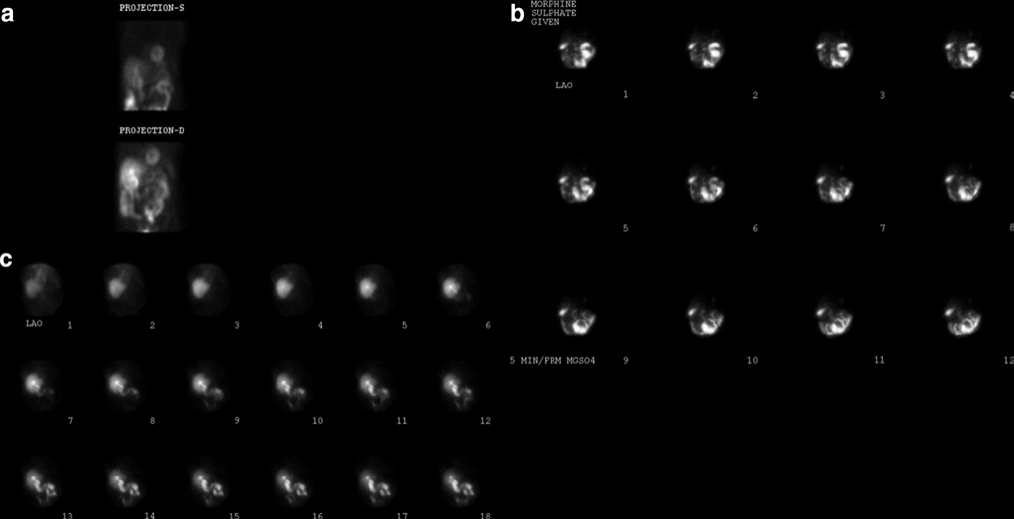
GB not visualized on the stress/rest MIBI (a) or HBS data (b), except post MS04 (c). *Incidentally, the MIBI, pre-MS04 and post-MS04 HBS studies were acquired in three different γ cameras*. GB, gall bladder; HBS, hepatobiliary scintigraphy; MIBI, methoxy isobutyl isonitrile.
